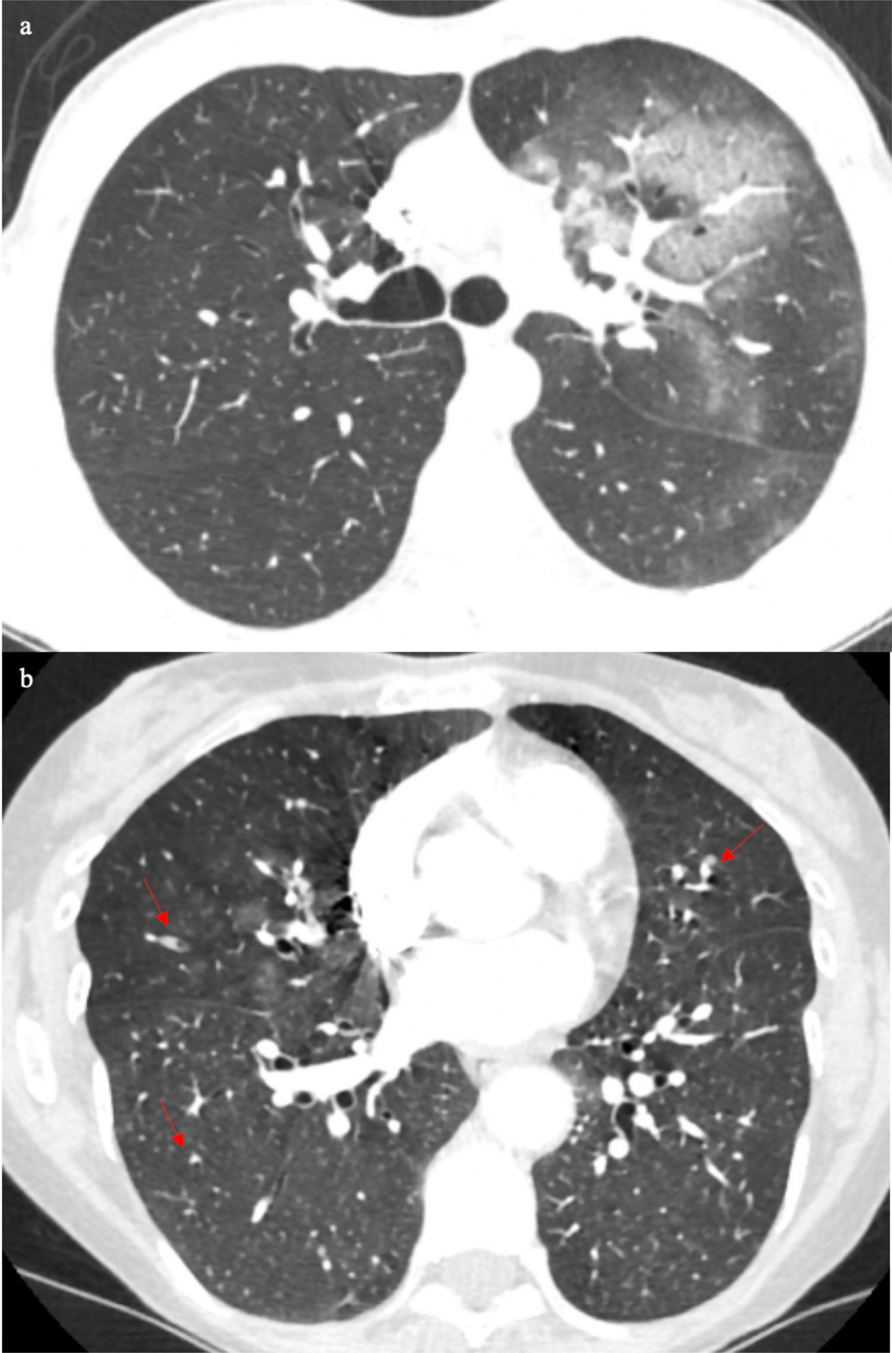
The CT showed predominantly left upper lobe central ground glass opacification (6a), which would favour lobar pneumonia, but COVID-19 infection is not completely excluded since it can also produce upper zone central ground glass opacification. Detailed inspection of the rest of the CT showed mucus plugging in the area of ground glass (Fig b, arrow), which would be consistent with bacterial infection.
